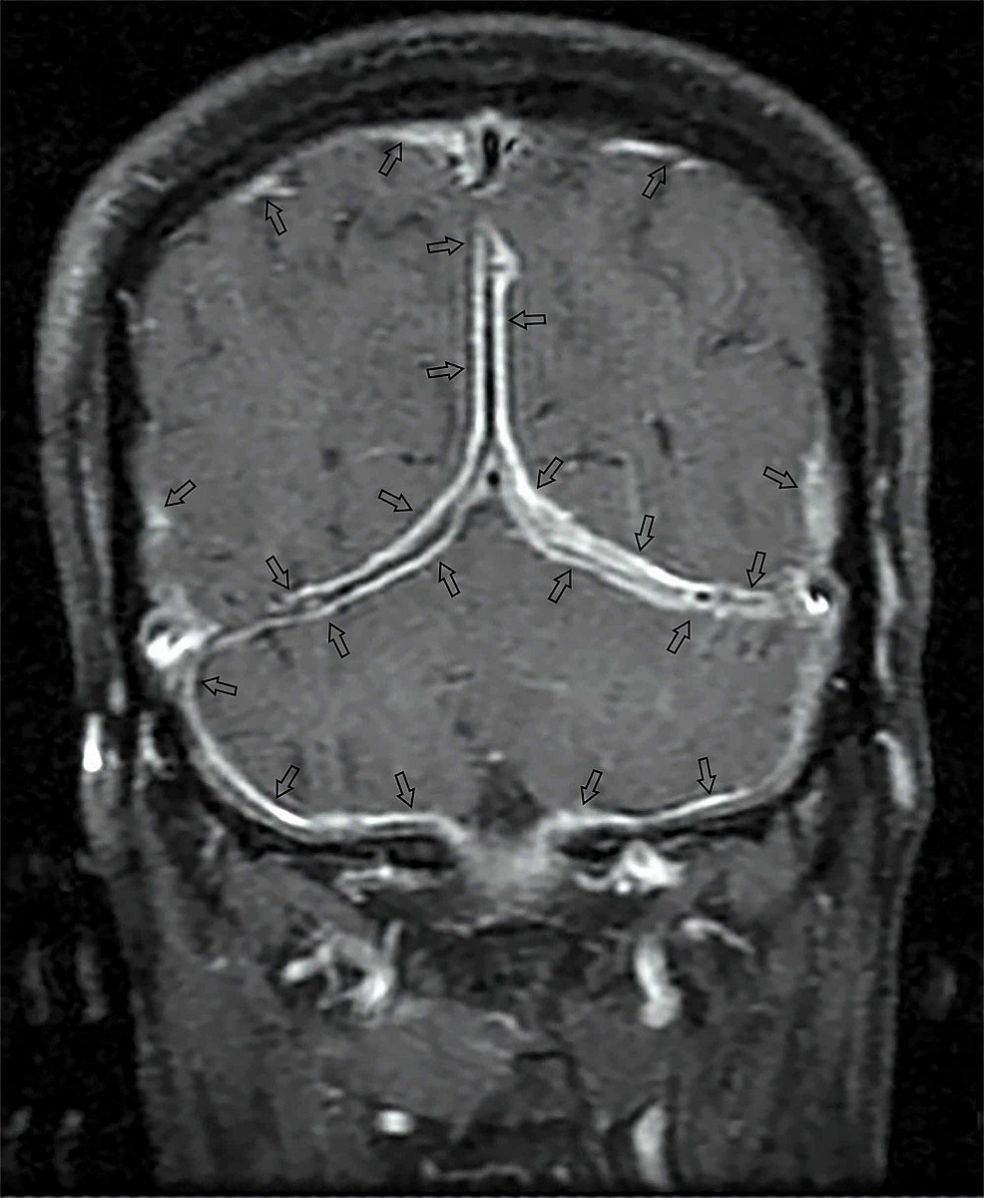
Pretreatment: Post-gadolinium coronal T1-weighted MRI showing diffuse posterior dural enhancement. Arrows show dural enhancement. MRI: magnetic resonance imaging
